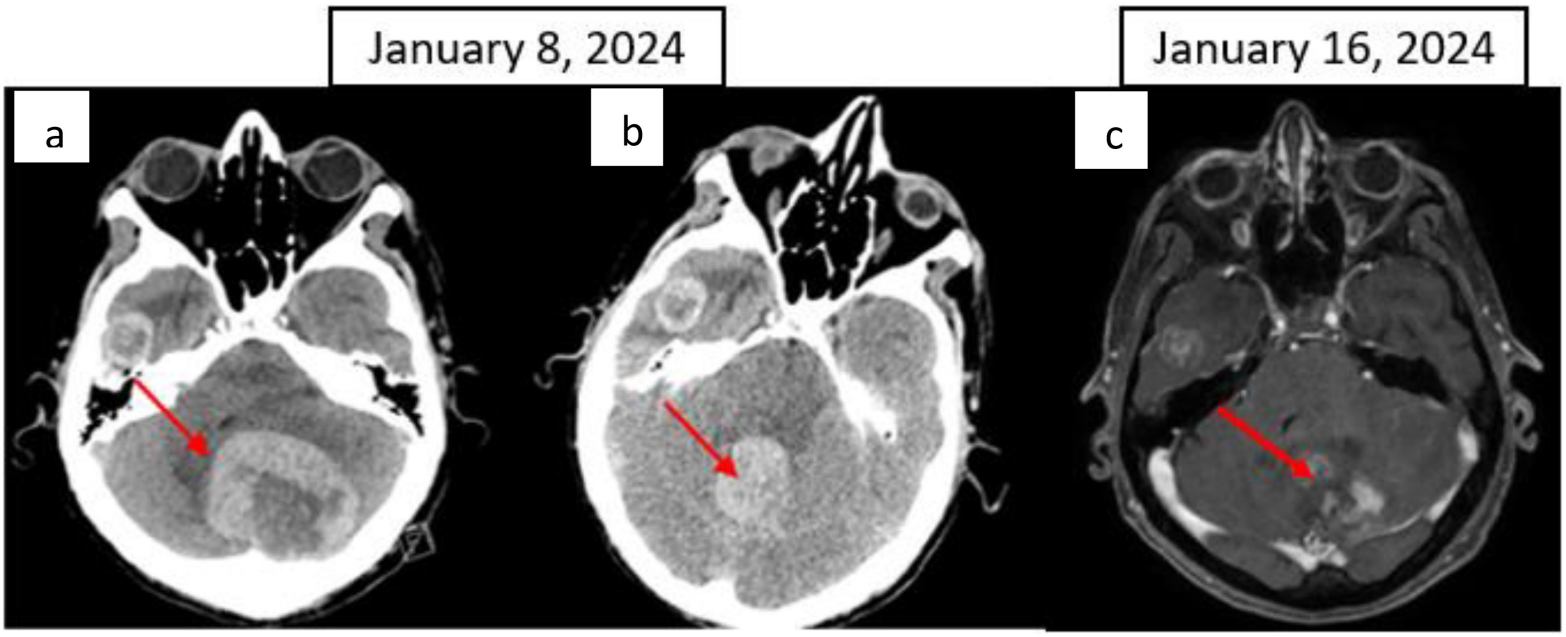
CT scan: (A) Left cerebellar metastasis measuring 55 × 51 mm; (B) right temporal metastasis with a necrotic centre measuring 24 × 18 mm; (C) Brain T1 MRI image after gadolinium injection, axial image. The red arrow indicates the cerebellar metastasis.
